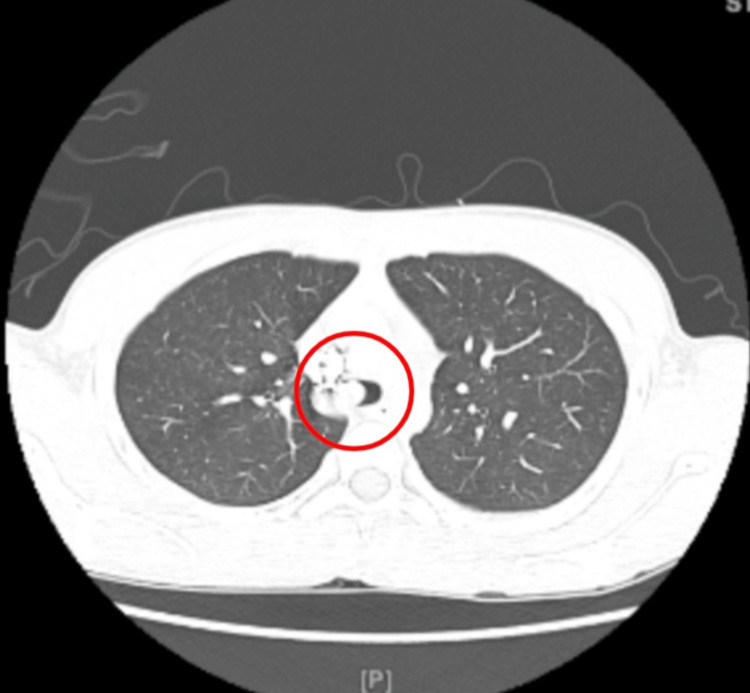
A computed tomography angiographic image with intravenous contrast depicting a soft tissue mass in the trachea measuring 19 millimeters (red circle). It did not demonstrate significant arterial enhancement.
